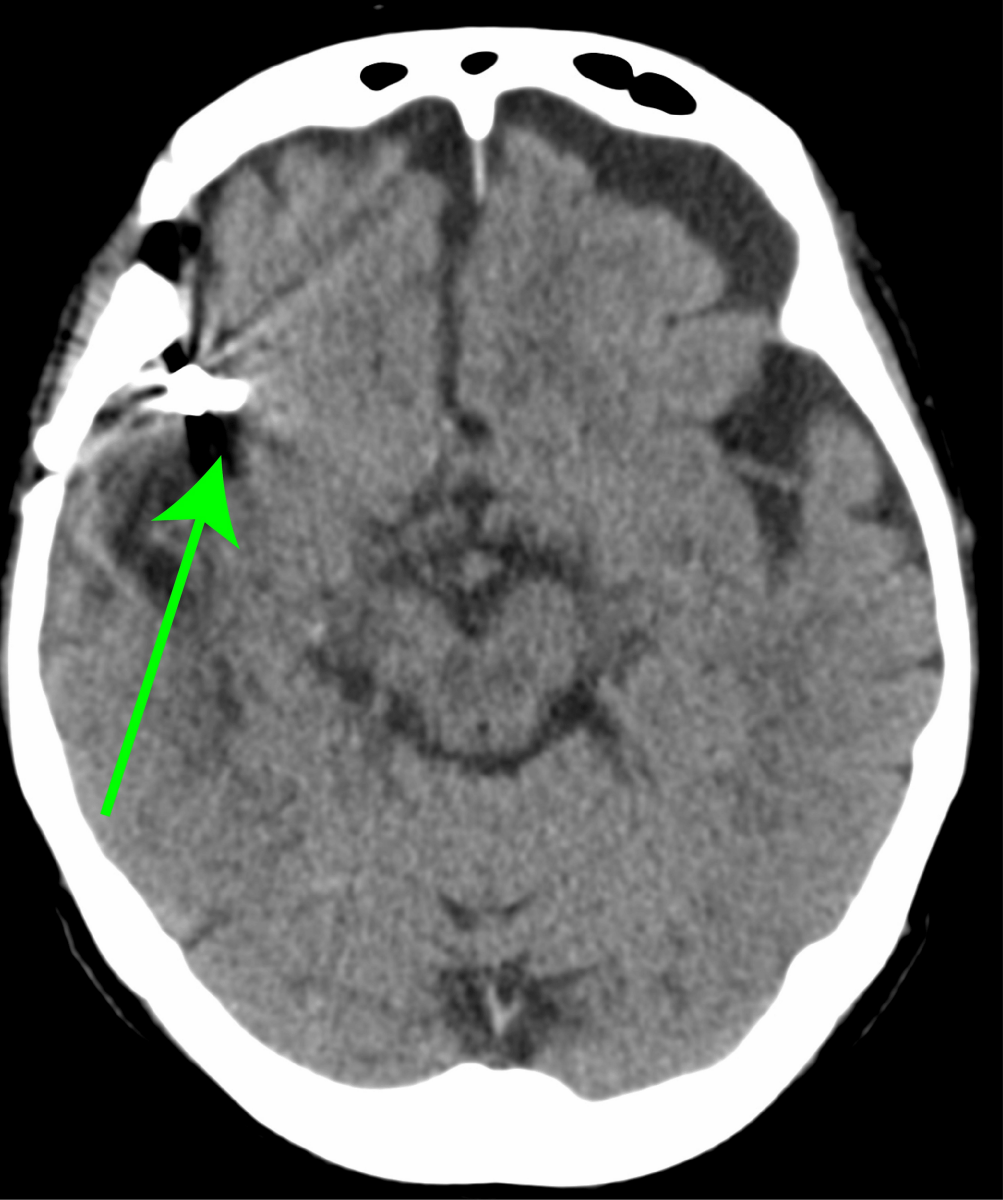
Brain computed tomography at the age of 53 years old. A clipping is observed in the right temporal lobe (green arrow).
